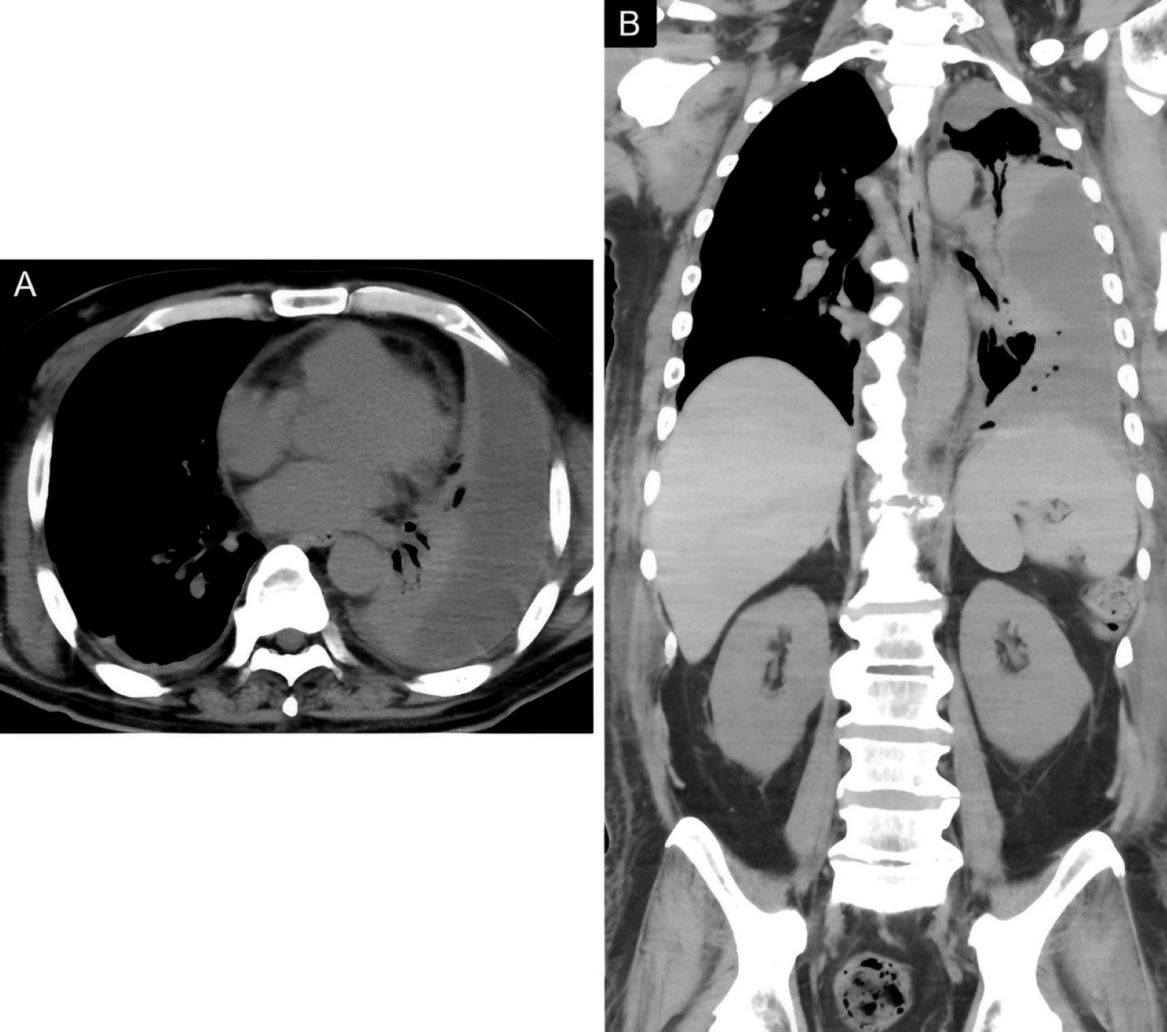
Non-contrast CT of the chest On day 21, left pleural effusion is depicted on CT, with values of 20 HU, associated with left lower lobe atelectasis (A, B). Note that small air bubbles are present in the fluid, which is consistent with pyothorax (B).
